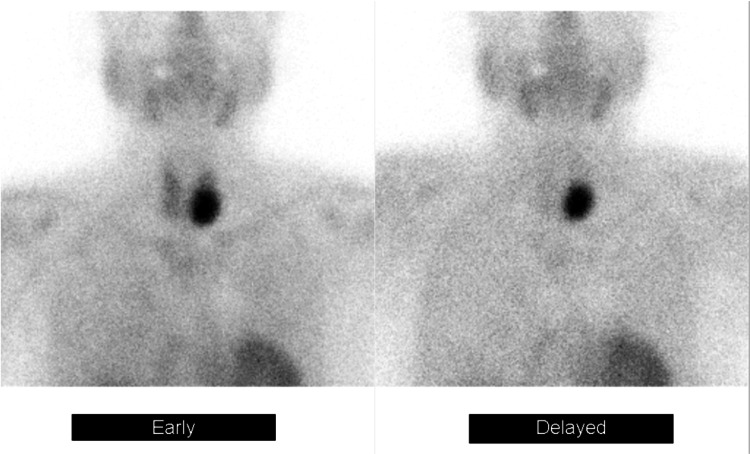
Tc-MIBI scintigraphy A nodular area demonstrating delayed uptake was observed near the left lobe of the thyroid. Tc-MIBI: technetium-99m methoxyisobutylisonitrile
